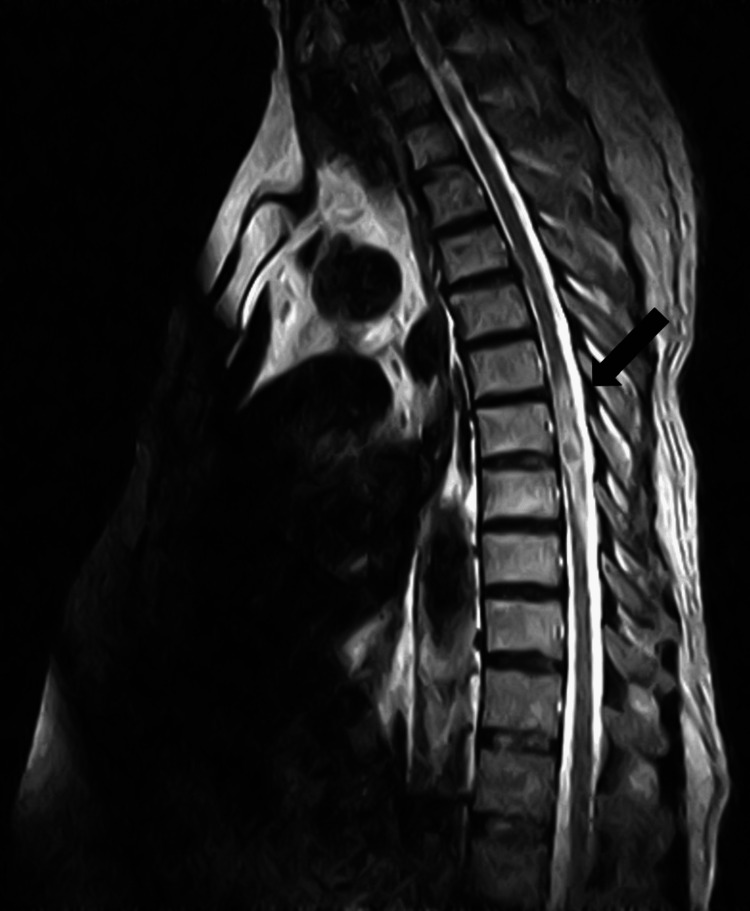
The sagittal T2-weighted thoracic MRI seen above showed a central low signal intensity lesion with a peripheral high intensity rim and surrounding edema in the cord at the T7/T8.
